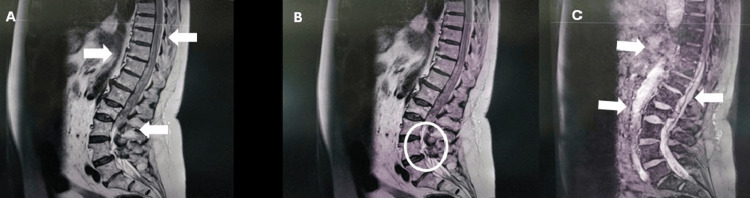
Sagittal MRI of the thoracolumbar spine demonstrating extensive spinal hematoma (A-B) Sagittal T1‑weighted images showing a large posterior epidural hematoma (white arrows) extending from T9 to L2, resulting in marked compression of the thecal sac with predominant involvement and anterior displacement of the conus medullaris. In B, the circled region highlights an inferior collection suggestive of a subdural component at L3-L4, associated with posterior deviation and crowding of the cauda equina nerve roots. (C) Sagittal T2‑weighted image confirming the craniocaudal extent of the epidural hematoma and the severity of resulting thecal sac compression (white arrows). Note the inferior high‑signal component corresponding to the suspected subdural extension. MRI: magnetic resonance imaging
